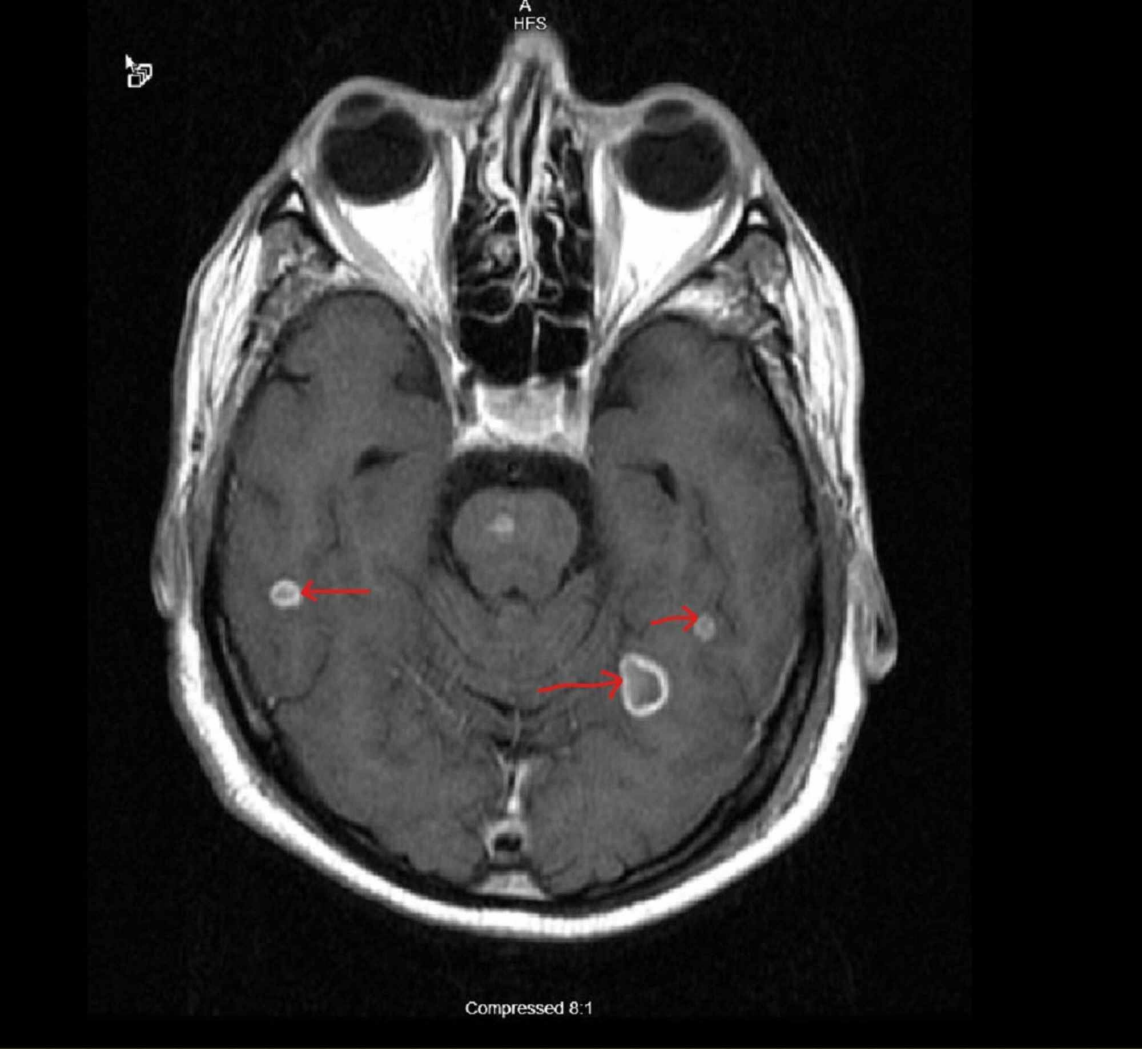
MRI brain different slice showing ring enhancing lesions in cerebral hemispheres (Arrows pointing)
